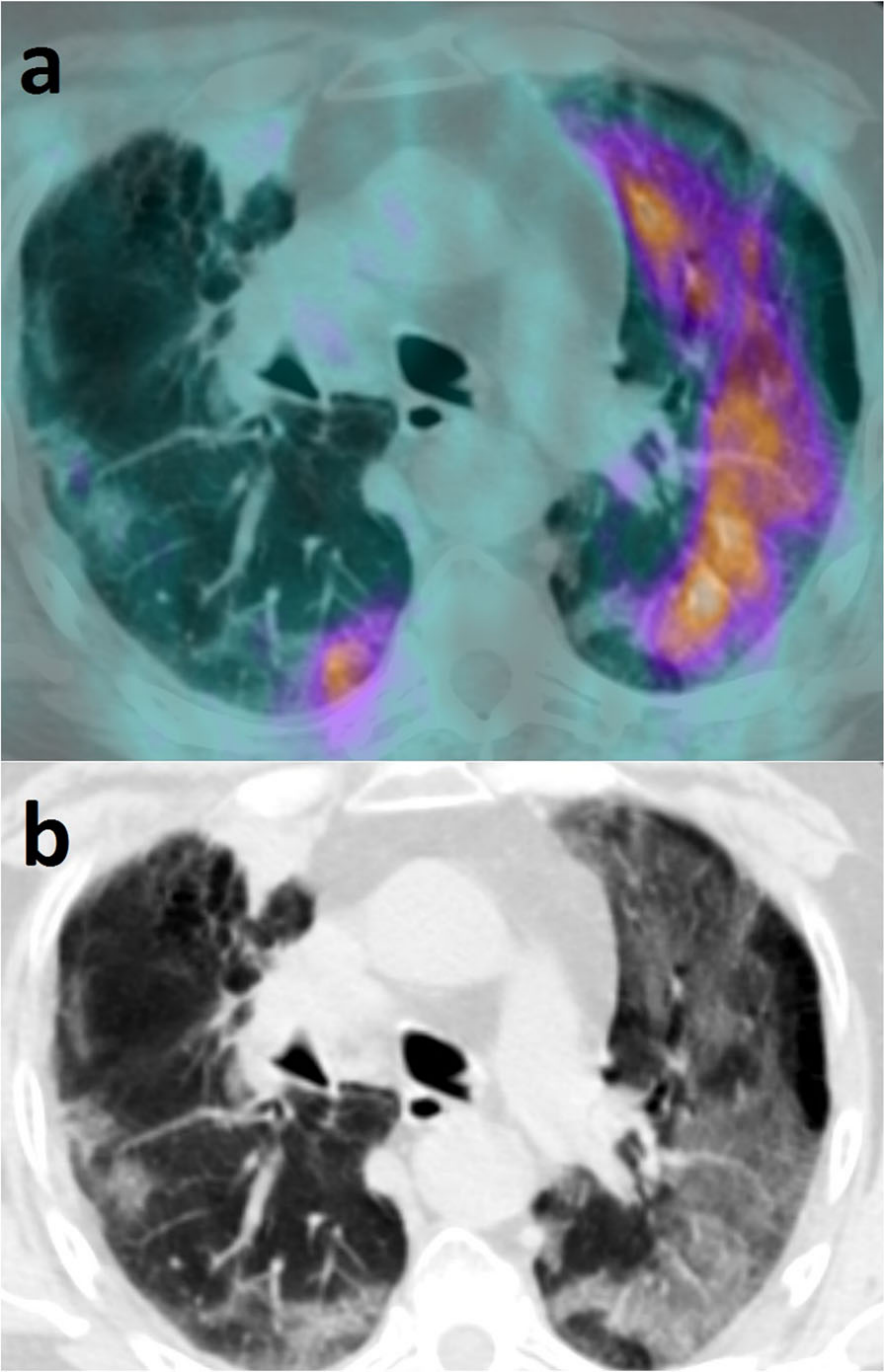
a Axial PET/CT view of the thorax showing diffuse, non-homogeneously increased FDG uptake (maximum SUV = 5) at the site of bilateral lung abnormalities. Additional images of pathologically increased FDG uptake were seen at the site of the primary tumor, in the apex of the right lung, and at the sites of multiple bone and liver metastases (not shown). No FDG-avid lymph-nodes were detected. b Corresponding CT view showing multifocal patchy centro-parenchymal and subpleural GGOs with subpleural fibrotic stripes of the right lung, as well as diffuse GGOs of the left lung with partial sparing of the subpleural space. Bronchial wall thickening and some bronchiectasis were also seen. No consolidations or micronodules, nor cysts or pleural effusion were detected. These features, in addition to the asymmetrical appearance and the sparing of the apical segments, orient, in first instance, towards an interstitial pneumonia of viral aetiology. In second instance, a pneumonitis induced by cisplatin-gemcitabine should be considered, as this is also characterized by multifocal or diffuse GGOs. Furthermore, although imaging features are not highly suspicious for *Pneumocystis Jirovecii*, the latter still represents a possible alternative diagnosis
